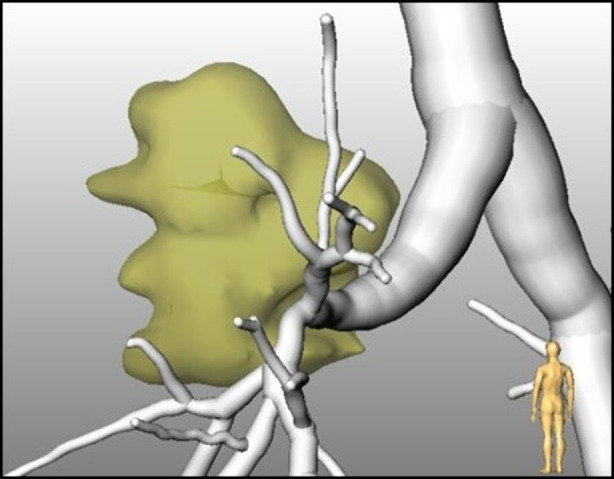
CT-based segmentation of a tumor in relation to the bronchial tree.
